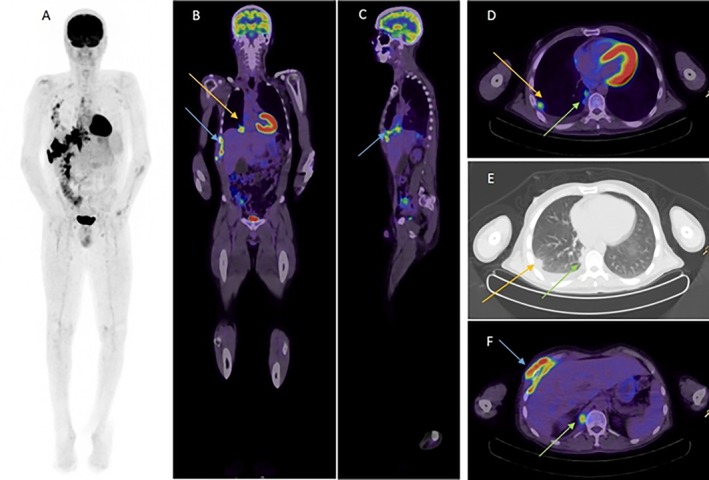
Image A shows whole body maximum intensity projection (MIP) used in PET imaging to visualise and represent a 3D distribution of radiotracer uptake in the body on a 2D plane. Image B, C, D and F are fused PET/CT images in the coronal, sagittal, transaxial and transaxial views respectively, whereas image E is a low dose CT transaxial view. Blue arrows demonstrate intense abnormal FDG uptake in the right hemithorax with involvement of the chest wall. Orange arrows demonstrate a 6 mm FDG avid right lower lobe lung nodule with associated right pleural effusion seen on the transaxial views. Green arrows demonstrate a FDG avid right distal para‐oesophageal node in the posterior mediastinum.
